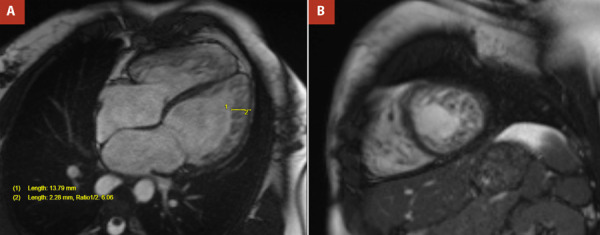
Cardiac MRI. Cine images in four chambers (A) and short-axis mid-ventricular (B) showing hypertrabeculation in the right and left ventricles.
